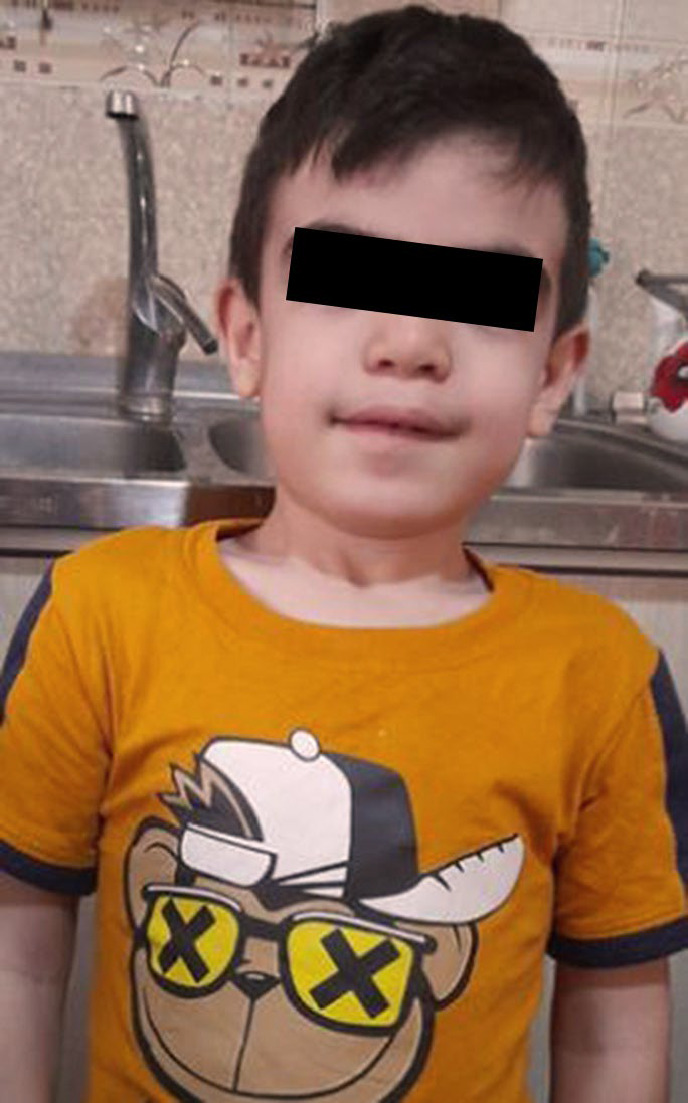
Male sibling axial T2 weighted brain MRI image shows periventricular
